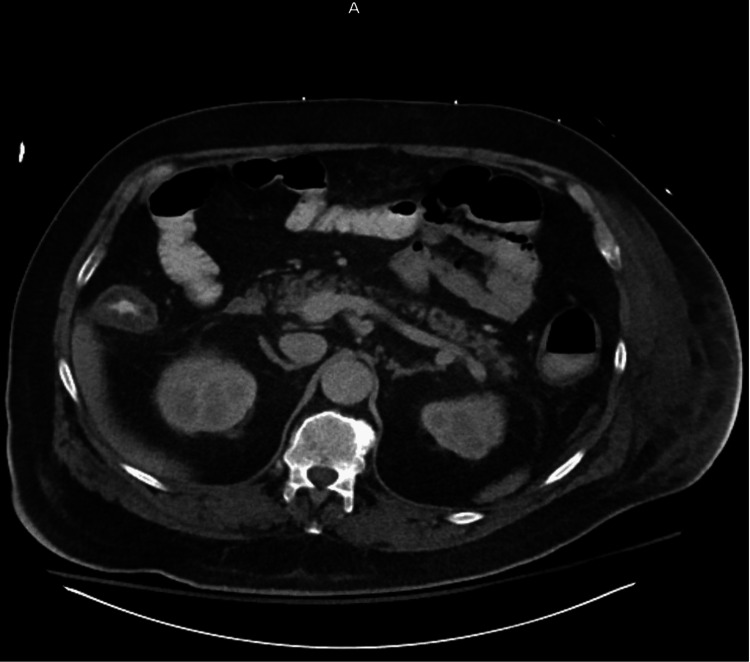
A follow-up CT scan of the abdomen and pelvis showed a normal-sized intestine with no pneumatosis intestinalis.
